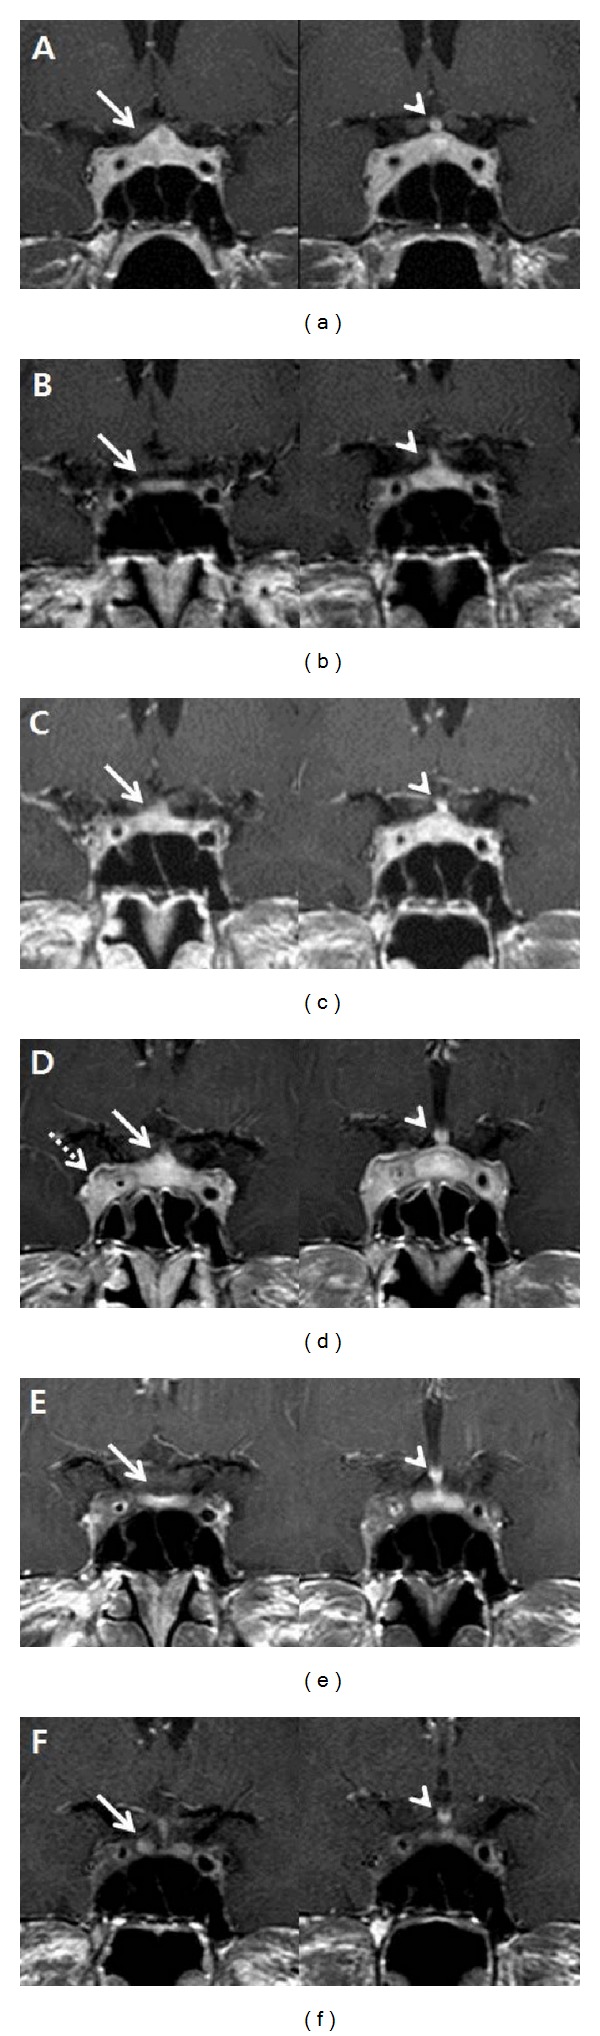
Serial follow-up sellar magnetic resonance image (MRI, contrast enhanced T1-weighted image): (a) initial contrast enhanced image showed suspicious mass of 8 mm in size at right pituitary (arrow, nonenhanced portion) and left sided stalk deviation with thickening (arrowhead). (b) Follow-up MRI obtained after 2 years of intermittent empirical steroid therapy for intractable headache showed reduction in the size of pituitary mass (arrow) and improvement of stalk deviation (arrowhead). (c) Follow-up MRI obtained 4 years after initial examination before gamma knife radiosurgery showed regrowth of pituitary mass with stalk deviation (arrow). (d) Follow-up MRI 1 year after gamma knife radiosurgery before high-dose steroid therapy showed remarkable enlargement of the pituitary mass with thickening and deviation of stalk (arrow) and invasion to cavernous sinus (dotted arrow). Significant stenosis of the right cavernous sinus internal carotid artery was present (arrowhead). (e) MRI obtained at 3 months after high-dose steroid therapy showed marked decline in the size of mass at pituitary gland and cavernous sinus (arrow). There was still visible luminal narrowing of cavernous internal carotid artery (arrowhead). There was also less soft tissue surrounding the right internal carotid artery. (f) MRI obtained at 48 months after high-dose steroid therapy showed reduction in the size of the pituitary and cavernous sinus mass (arrow). Thickening of the pituitary stalk was also decreased (arrowhead), and the soft tissue surrounding the right internal carotid artery was improved.
